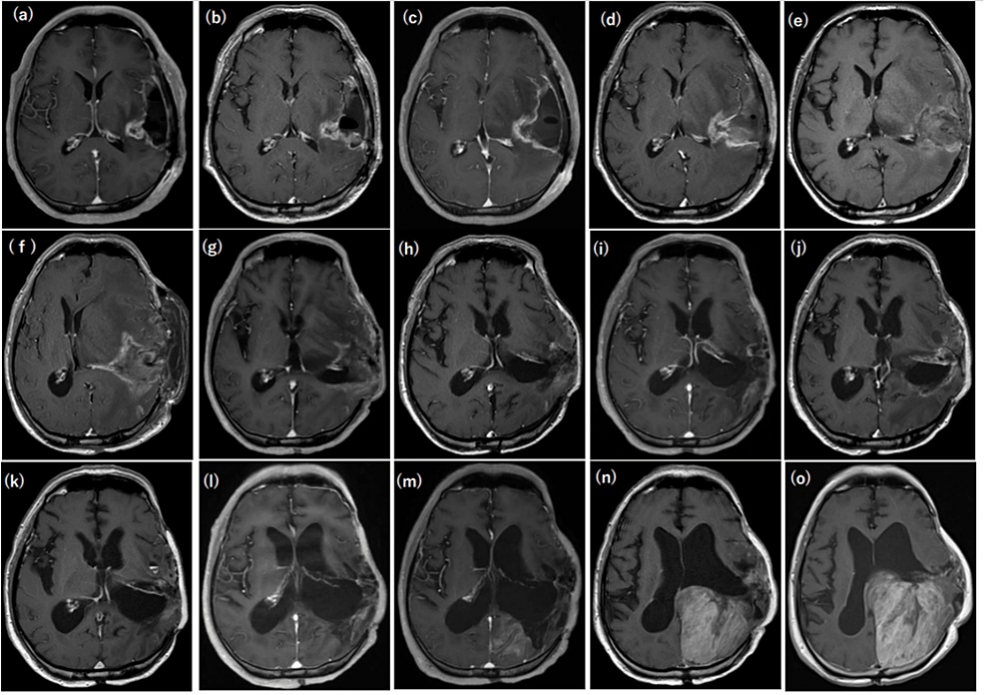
Contrast-enhanced T1-weighted axial brain MRI before, during, and after boron neutron capture therapy (BNCT). (a) One day before BNCT. (b) One week after BNCT. (c) One month after BNCT. (d) Three months after BNCT. (e) Six months after BNCT. Contrast-enhanced lesions appeared, but it was unclear whether these lesions were due to necrosis or recurrence. (f) Eight months after BNCT. The lesion was determined to be due to necrosis, and the necrotic lesion excision was performed. (g) 12 months after BNCT. (h) 18 months after BNCT. (i) 24 months after BNCT. (j) 30 months after BNCT. (k) 36 months after BNCT. (l) 48 months after BNCT. (m) 51 months after BNCT. A contrast-enhanced lesion appeared on the dorsal side of the irradiation field. (n) 54 months after BNCT. (o) 58 months after BNCT.
